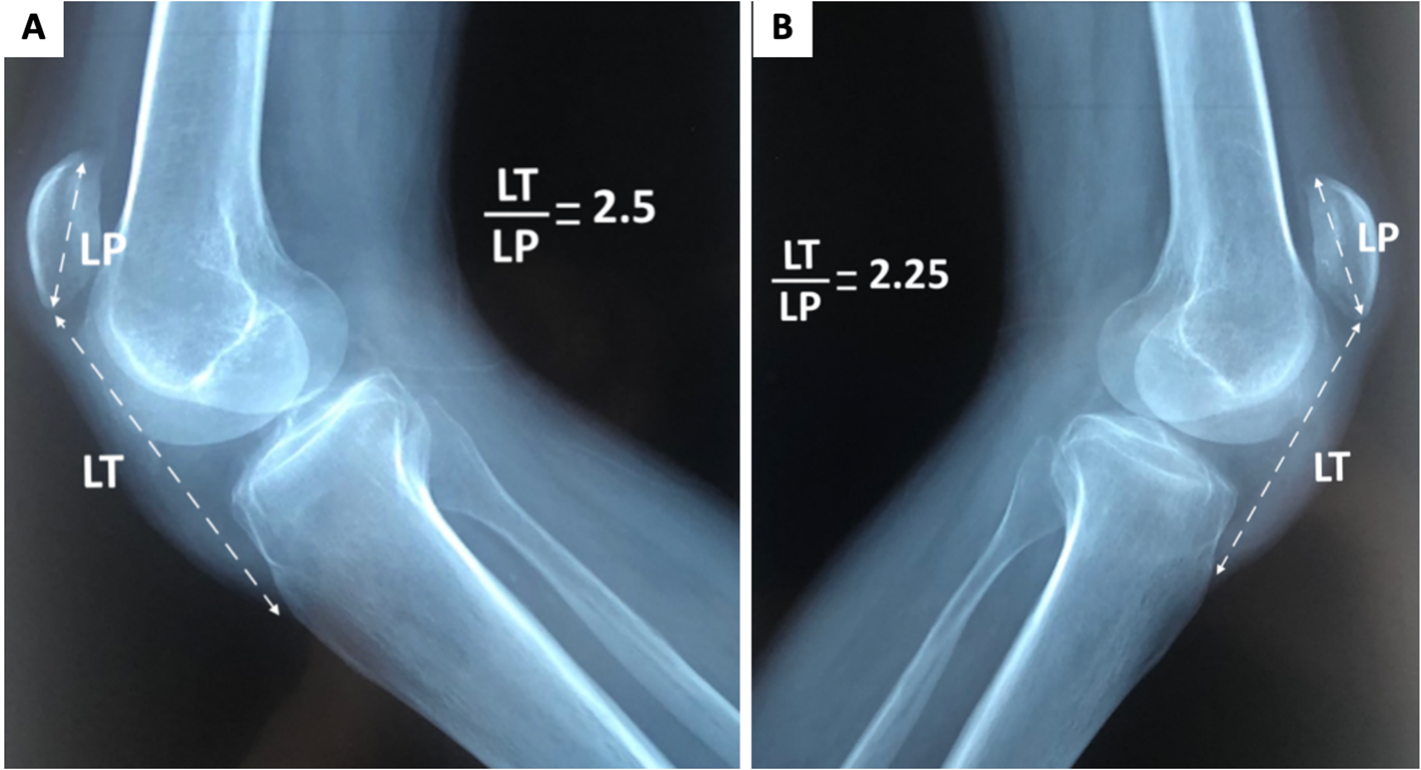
Conventional radiography findings in both knees (a: right knee, b: left knee). Bilateral aspect of patella alta with the Insall-Salvati ratio. LP: length of the patella; LT: length of the patellar tendon
